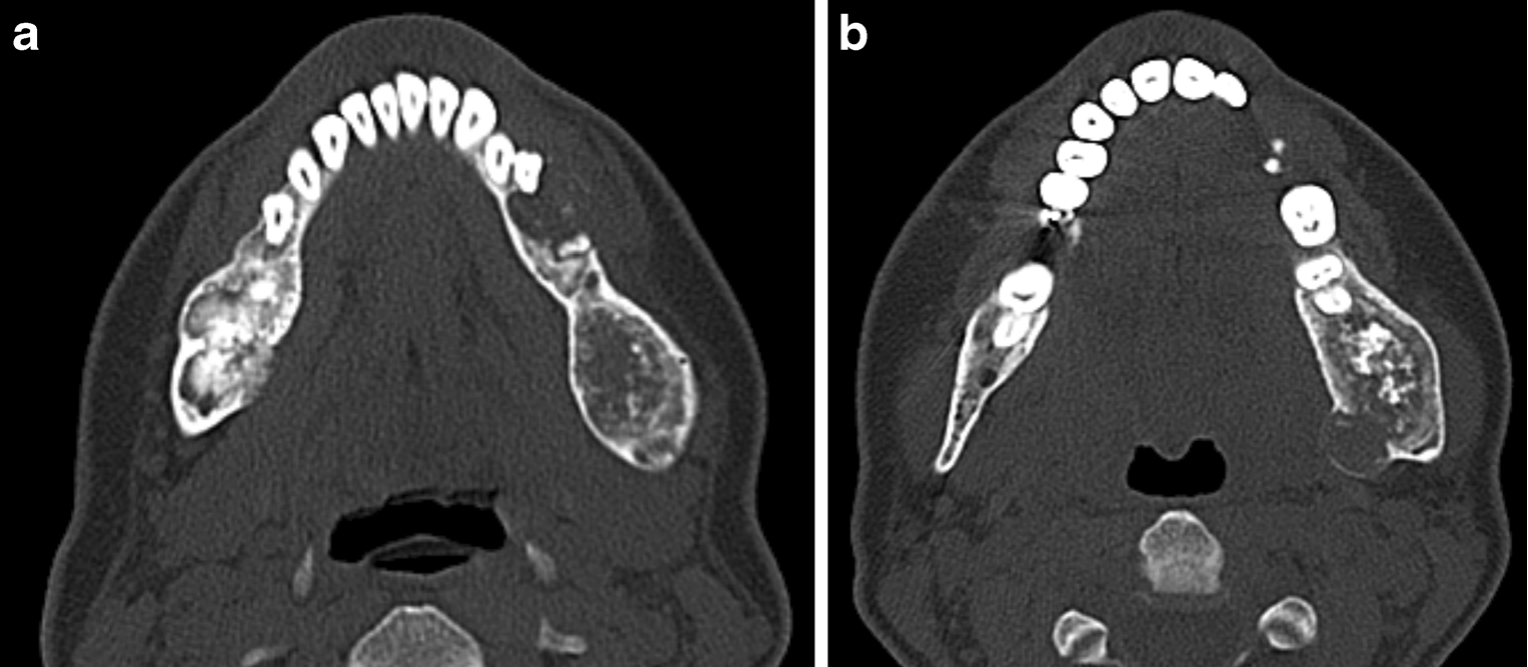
a), b) Axial 1.5 mm CT images through the mandible of a 30-year-old female with HPT-JT. There are multiple bilateral ossifying fibromas of the mandible, as is typical of the condition. Ossifying fibromas are composed of fibrous tissue with varying amounts of mature bone, and; therefore, as demonstrated here, some lesions are purely lucent, while others will have a more conspicuous calcified componentOssifying fibromas of the jaw are benign lesions with the risk of malignant degeneration being less than <0.5 % [5]. However, they demonstrate locally invasive features, e.g., they may splay the teeth [4]. They are expansile and can cause facial asymmetry. They can extend into the maxillary sinus (Fig. 3). Extension into the orbital floor may precipitate periorbital swelling, orbital pain or changes in visual acuity (Fig. 4). If left untreated, ossifying fibromas may continue to enlarge and become severely disfiguring (Fig. 5).
